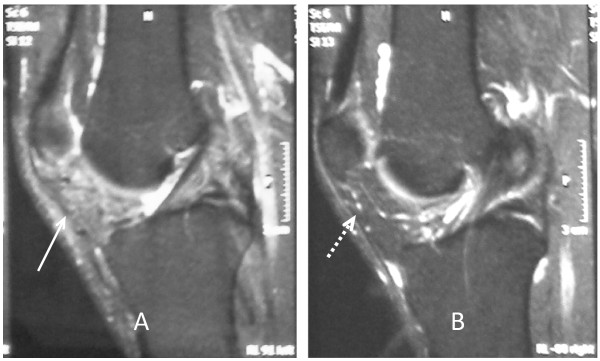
Postoperative follow-up MRI of both knees. (A) Magnetic resonance imaging (MRI) of left knee six months after surgery shows the absence of cystic lesion with a mild increase of signal intensity of Hoffa's fat pad (arrow). (B) Right knee MRI shows a normal appearance and signal intensity of the infrapatellar fat pad (dashed arrow).
